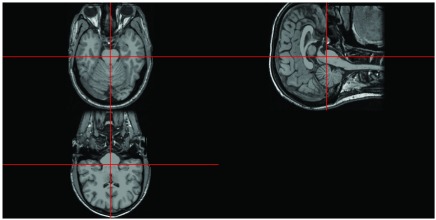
Plot of T1-weighted image from bert subject in Freesurfer.
